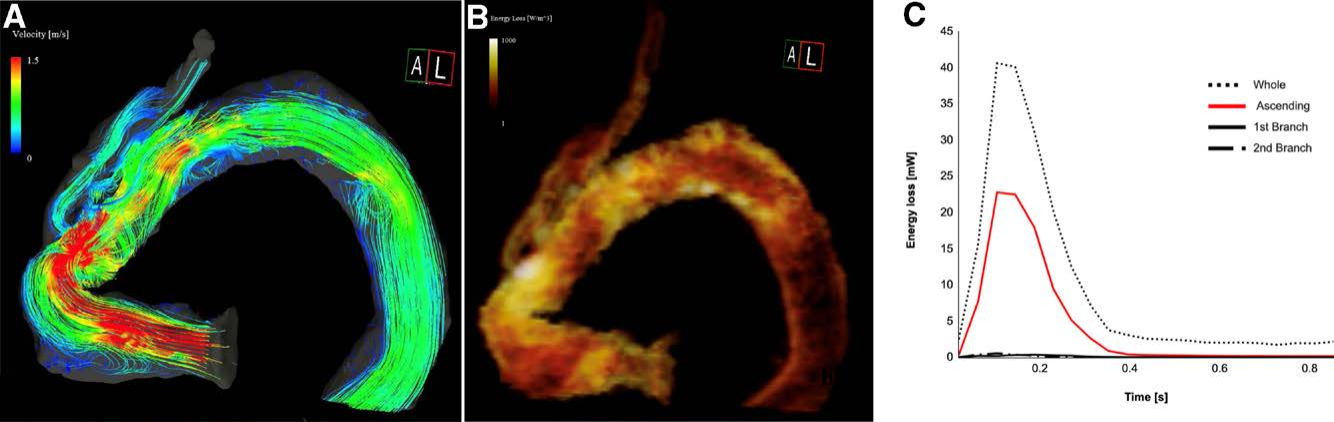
Four-dimensional flow MRI analysis of the streamlines and VEL before the revision surgery. (A) An acceleration flow is observed around the kinked lesion in the acceding portion of the graft in the streamlines. (B) The elevated VEL is represented by the bright area in the middle image. (C) The graph shows that the whole VEL was elevated. The VEL at the ascending portion was higher than that in the cervical branches. MRI = magnetic resonance imaging, VEL = viscous energy loss.
